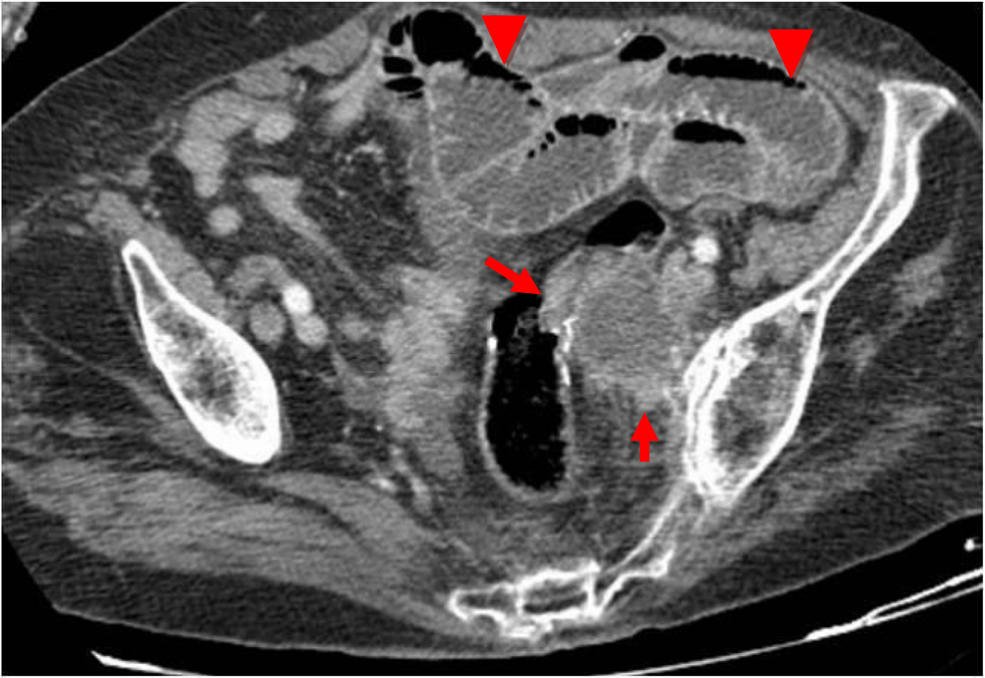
Contrast-enhanced pelvic CT of a 79-year old woman with obstructive symptoms who had undergone sigmoidectomy for adenocarcinoma 1 year earlier. There is asymmetric thickening of the bowel wall and a hypodense mass adjacent to the colorectal anastomosis (*arrows*), causing proximal bowel distension (*arrowheads*), confirmed as tumor recurrence at surgery
